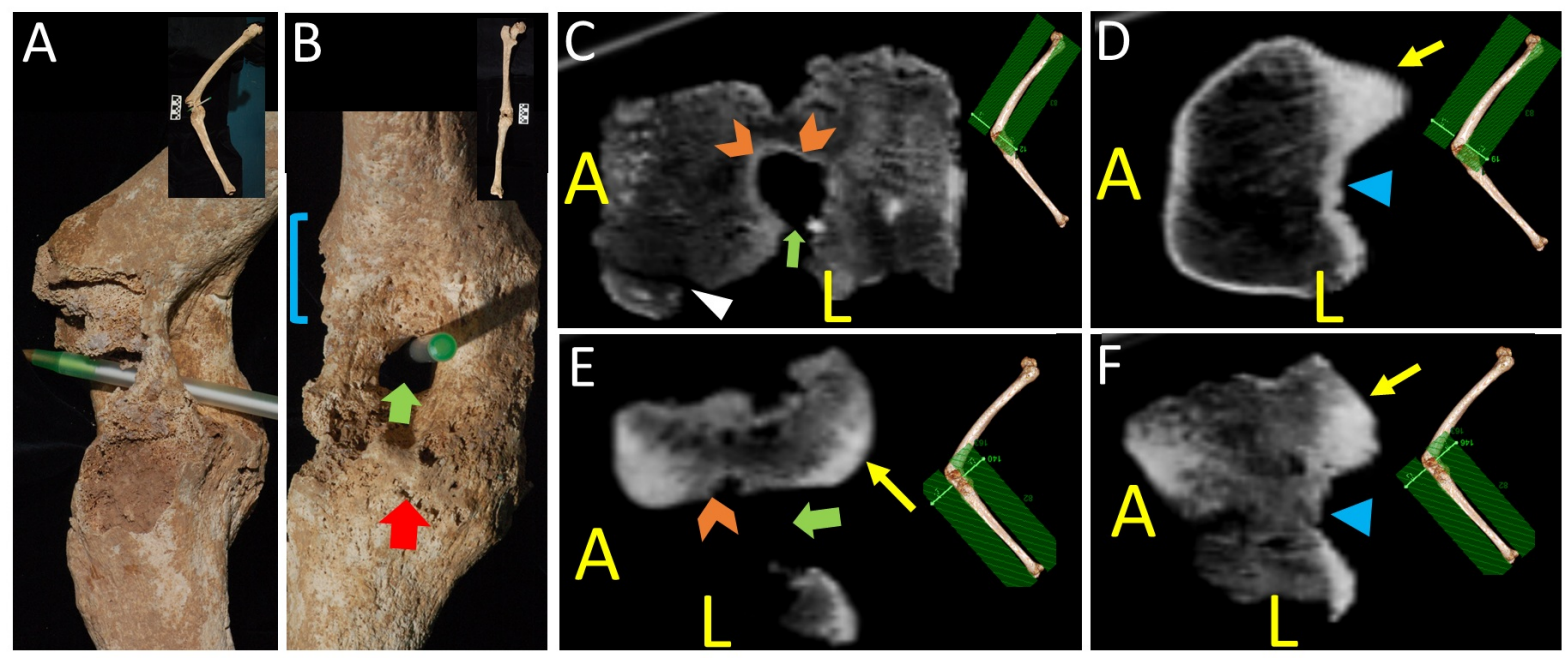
Views of Philip’s Leg A: Lateral view of the knee joint with a pen demonstrating the persistent space from the wound tract and foreign body granuloma and surrounding ankylosis. B: Posterior view of the knee joint along with the persistent space from the wound and foreign body granuloma and surrounding ankylosis. C: Axial CT scan through the injured joint space oriented perpendicular to the long axis of the femur. White triangle arrowheads point to areas of post-mortem damage identified by lack of compact bone on the surface and fracture lines separating continuous channels in the cancellous bone. Orange chevron arrowheads demonstrate reactive sclerotic bone on the walls of the granuloma. Note the osteolytic nature of the lesion with some sclerotic changes around the hole typical of lesions resulting from foreign body granulomas. D: Axial CT scan through the femoral condyles oriented perpendicular to the long axis of the femur. The yellow arrow represents normal cortical bone on the surface of the femoral condyles, whose shape can be appreciated. E: Axial CT scan of the injured joint space at a level inferior to the intercondyloid fossa oriented perpendicular to the long axis of the tibia. The hole can be seen along its major axis (green arrow). The difference between the abnormal bone lining the hole (orange chevron) and the normal cortical bone along the inferior limit of the femoral condyle (yellow arrow) can easily be distinguished. F: Axial CT scan of the joint space at the level of the intercondyloid fossa oriented perpendicular to the long axis of the tibia. The yellow arrow represents normal cortical bone on the femoral condyles, whose shape can be appreciated. Note that the bone surrounding the intercondyloid fossa is evenly corticated, differentiating it from the granuloma space. Labels - Brackets: femoral condyles, Red arrow: Tibial plateau, Green block arrow: wound tract, Blue triangle arrow: Intercondyloid fossa, A: Anterior, L: Left
